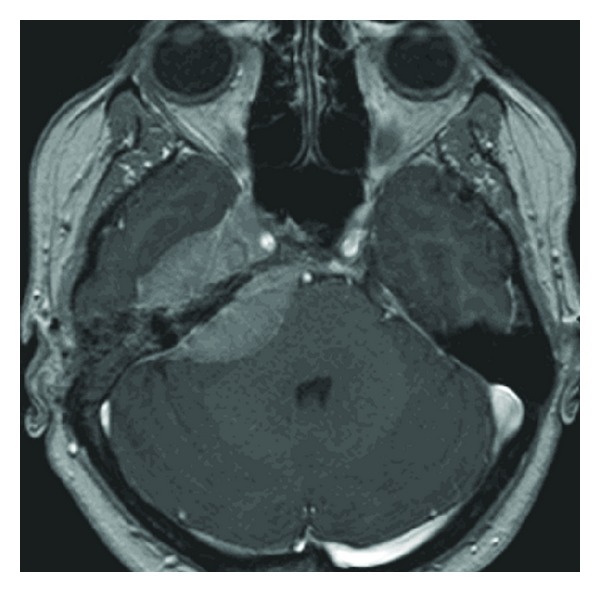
Axial, T1-weighted magnetic resonance imaging scans with gadolinium contrast, showing a right enhancing meningeal lesion involving the anterior and the posterior part the petrous apex with the characteristic meningeal tail.
